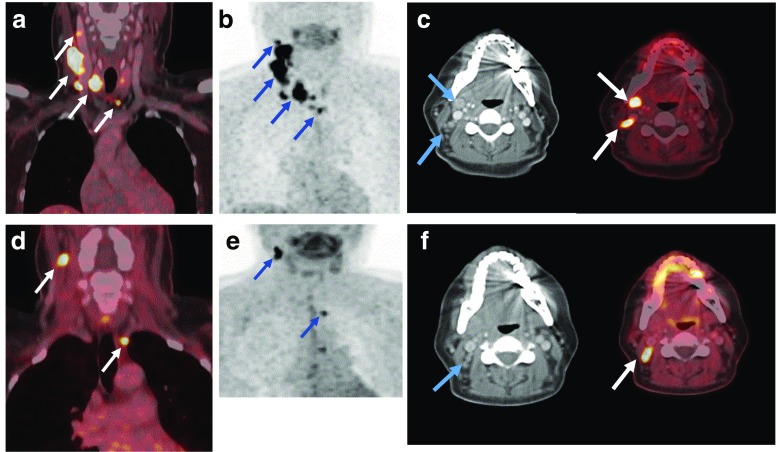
A 47-year-old female patient with further increasing basal calcitonin levels after surgery. a–c Preoperative [^18^F]DOPA PET/CT images show a DOPA-positive primary tumour in the right thyroid lobe with multiple DOPA-positive lymph nodes in the right cervical region (levels II, III, IV and V) as well as in the lower central region (level VI). d–f Postoperative [^18^F]DOPA PET/CT images in the same patient show persistently DOPA-positive lymph nodes in the right upper accessory level V and central level VI
